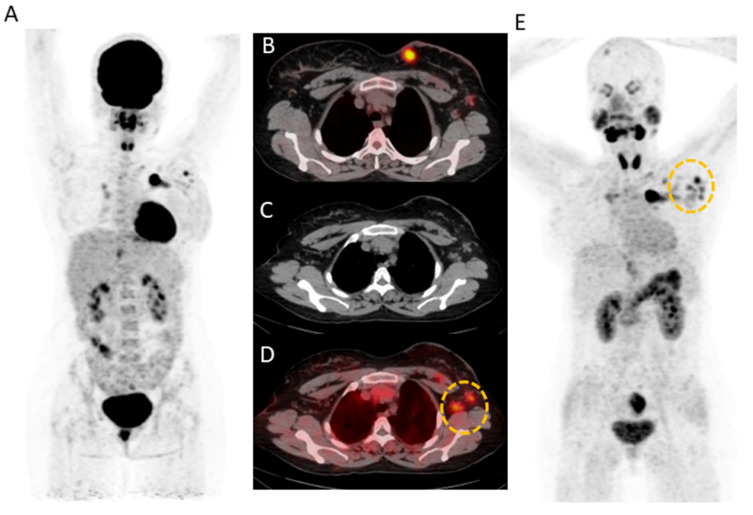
(A) 31-year-old female underwent modified radical mastectomy (ER+/PR+/Her2-) and 2 cycles of docetaxel chemotherapy. [^18^F]F-FDG (A,B) and [^68^Ga]Ga-DOTA.SA.FAPi (E) avid irregular mass (measuring 2.6 × 2.4 cm) noted in the breast upper inner quadrant. [^68^Ga]Ga-DOTA.SA.FAPi showed increased radiotracer uptake noted in the left axillary level I and II lymph node (D) corresponding to the CT (C) as compared to that of [^18^F]F-FDG (yellow circle). [^18^F]F-FDG shows diffuse non-specific radiotracer uptake in the entire axial skeleton, which is likely reactive (A).
